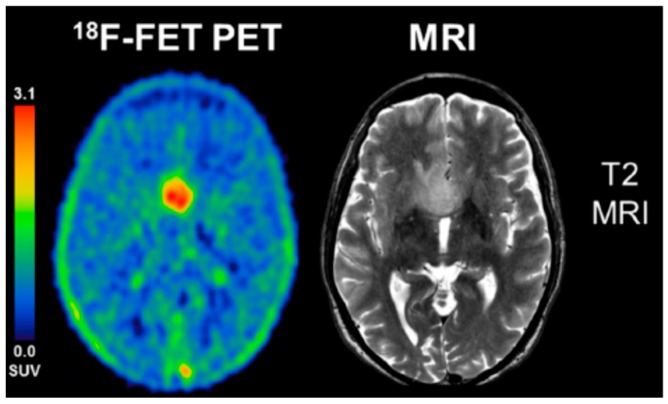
^18^F-FET PET summation image from 5–15 min is contrasted to a T2 MR image in this patient with a WHO III astrocytoma, IDH-wild-type, without 1p/19q co-deletion. This was a non-enhancing tumor on MRI. Note excellent tumor to background contrast for the FET PET image, with standard uptake values (SUV) of up to 3. Adapted from Unterrainer et al. *Eur. J. Nucl. Med. Mol. Imaging* 2018, 45, 1242 with permission [51].
